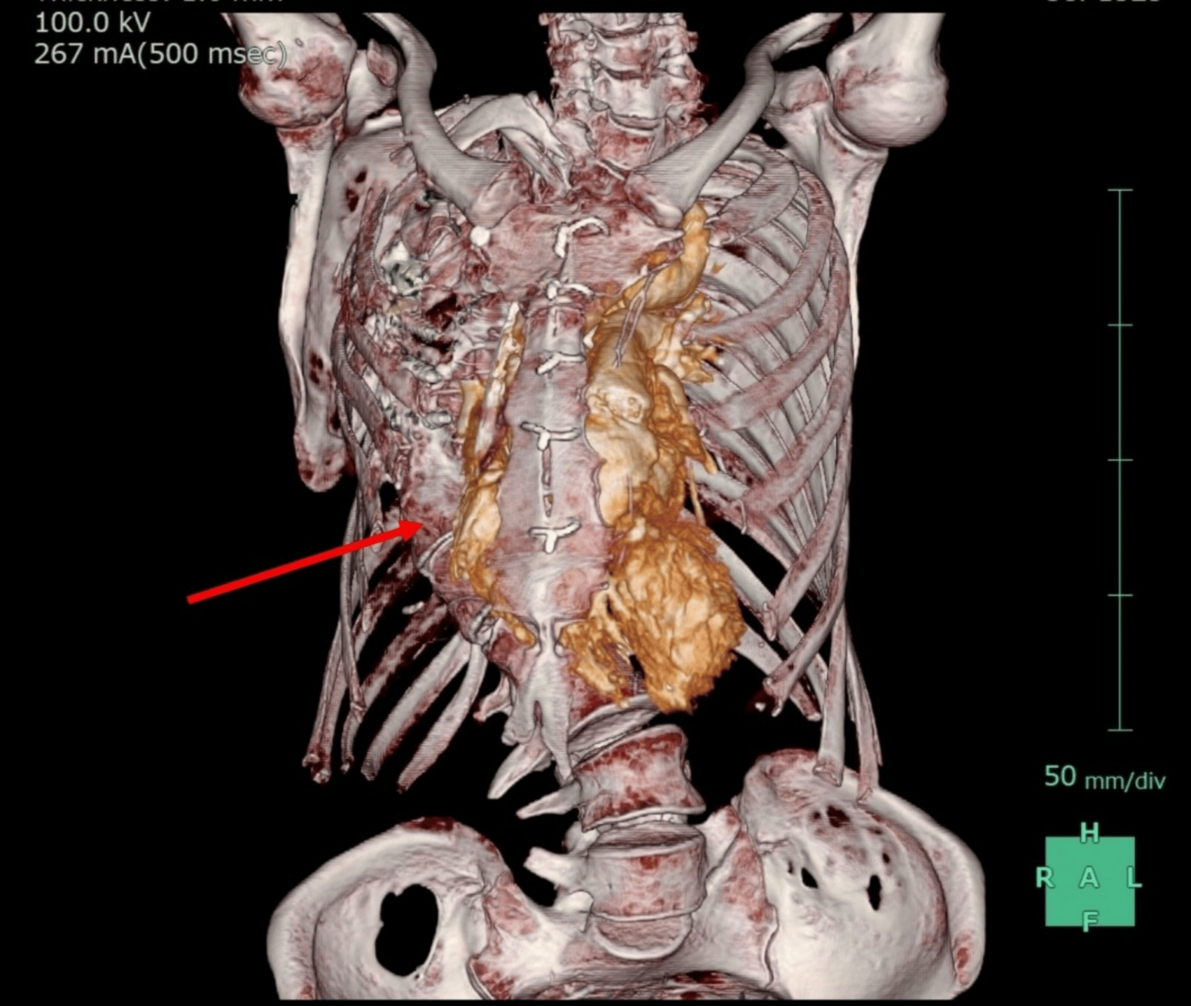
Three-dimensional reconstruction generated from CT imaging The red arrow indicates the presence of severe scoliosis.
